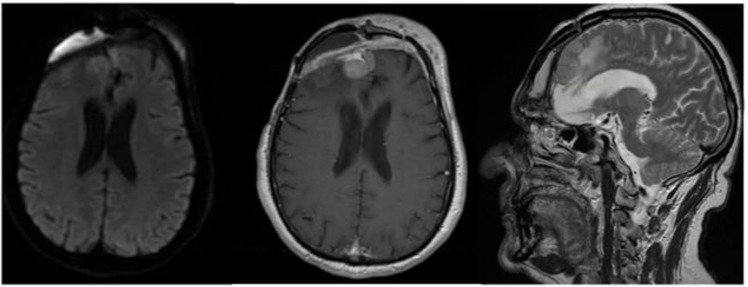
Brain MRI with evidence of a right frontal epidural empyema and meningioma recurrence (left, DWI; center, T1-weighted with contrast; right, T2-weighted).
